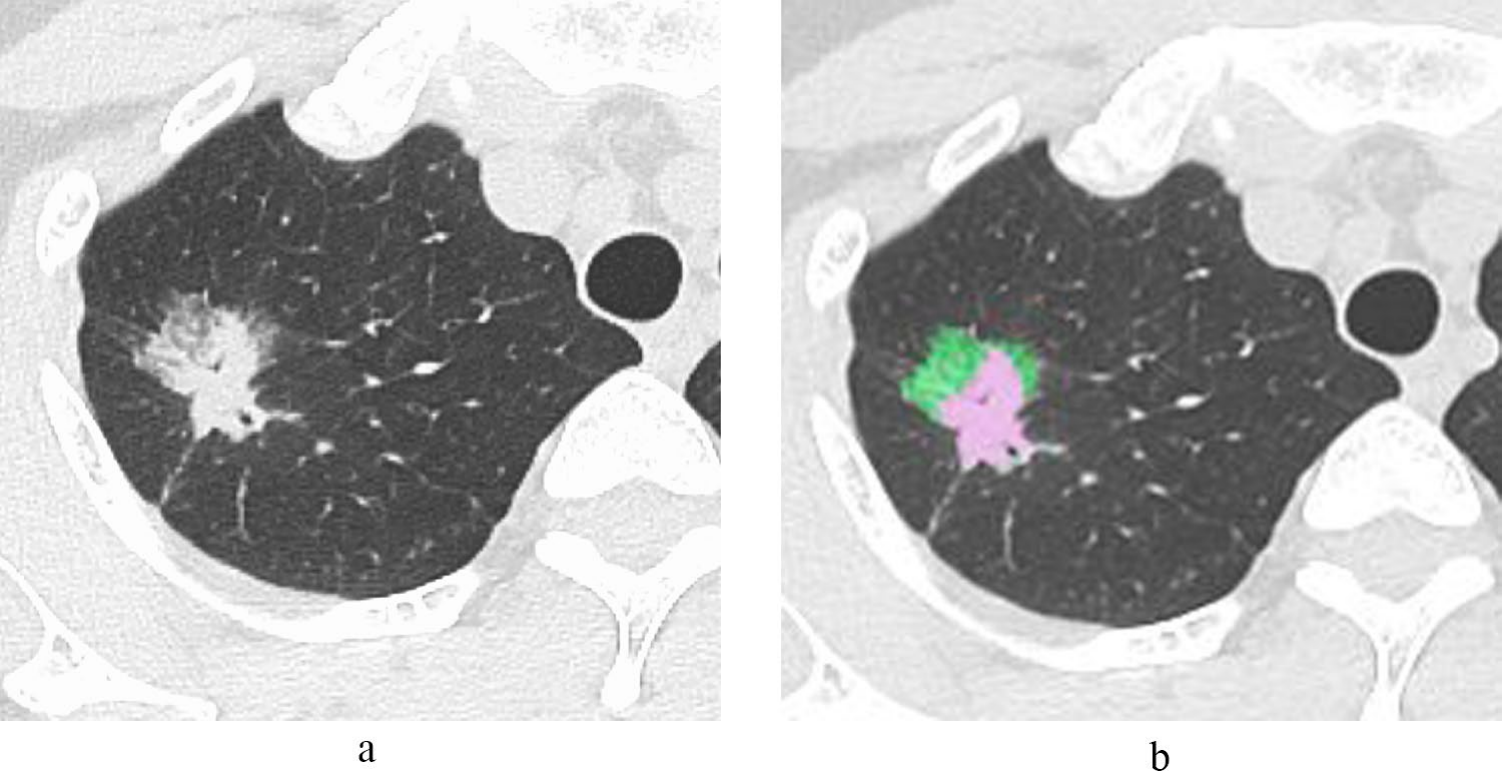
A 46-year-old woman with invasive adenocarcinoma (TPS = 0%). a HRCT shows a part-solid nodule. The diameters of the tumor and solid component are 28.7 mm and 19.1 mm, respectively. The rate of the solid component diameter is 66.6%, b The volume of the tumor and solid component are 5752.3 (pink and green area) mm^3^ and 3301.6 mm^3^ (pink area), respectively. The rate of the solid component is 57.4%. *TPS* tumor proportion score, *HRCT* high-resolution CT
